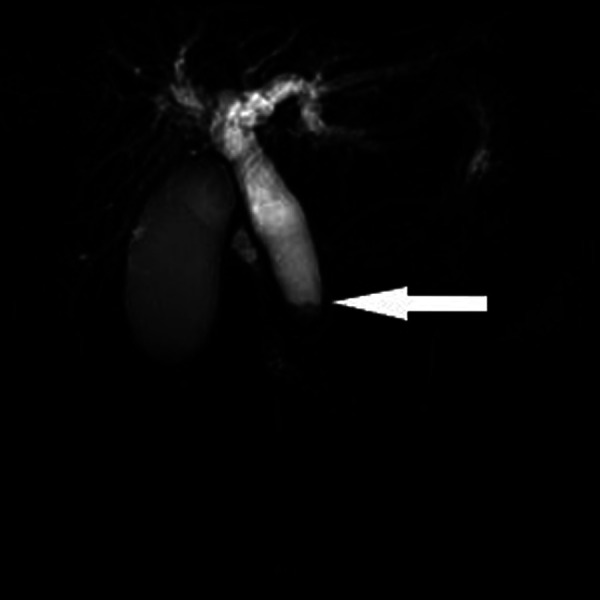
Magnetic resonance imaging showing complete obstruction of the distal bile duct (white arrow).
